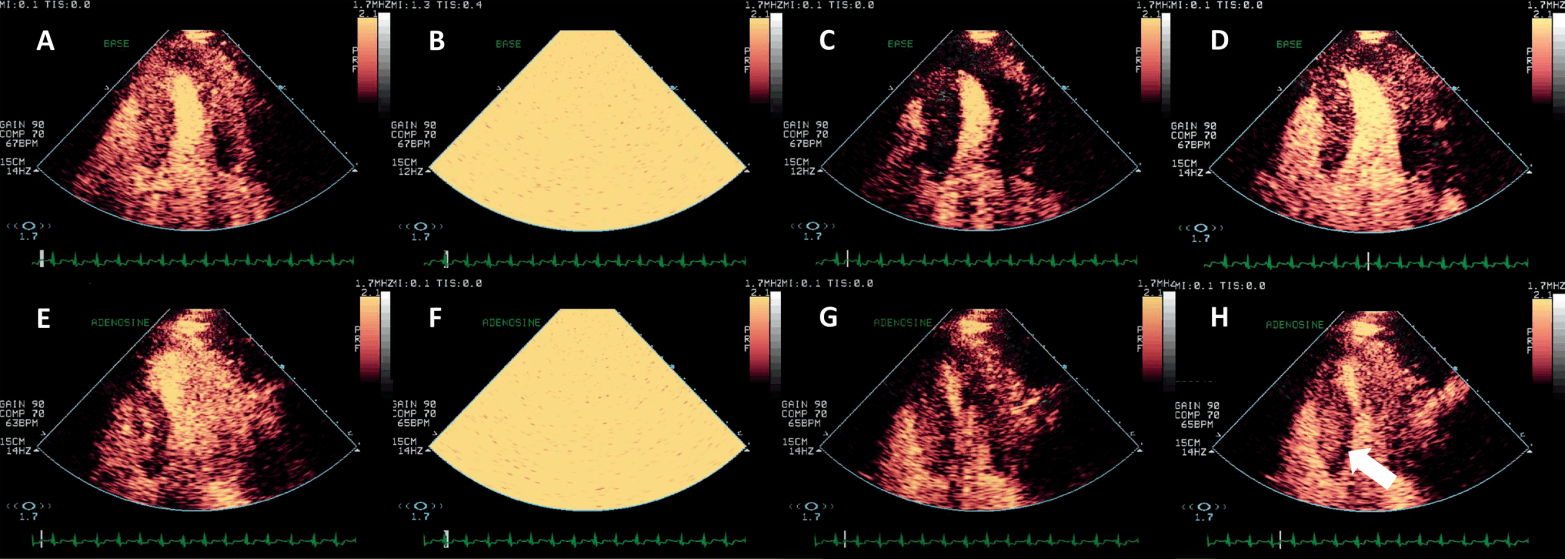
Vasodilator stress echocardiography with Adenosine, using a
